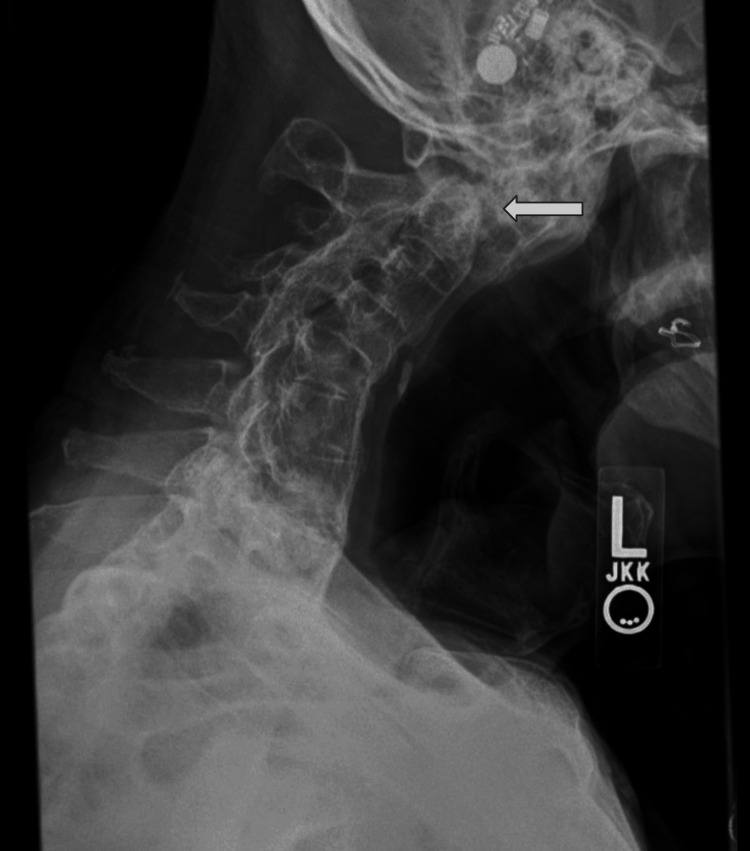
Preoperative lateral view X-ray of the dens fracture, bilateral C2 facet fracture, and lateral mass fractures with C1-C2 stenosis (white arrow) Note the dens fracture and ankylosed cervical spine and kyphosis
